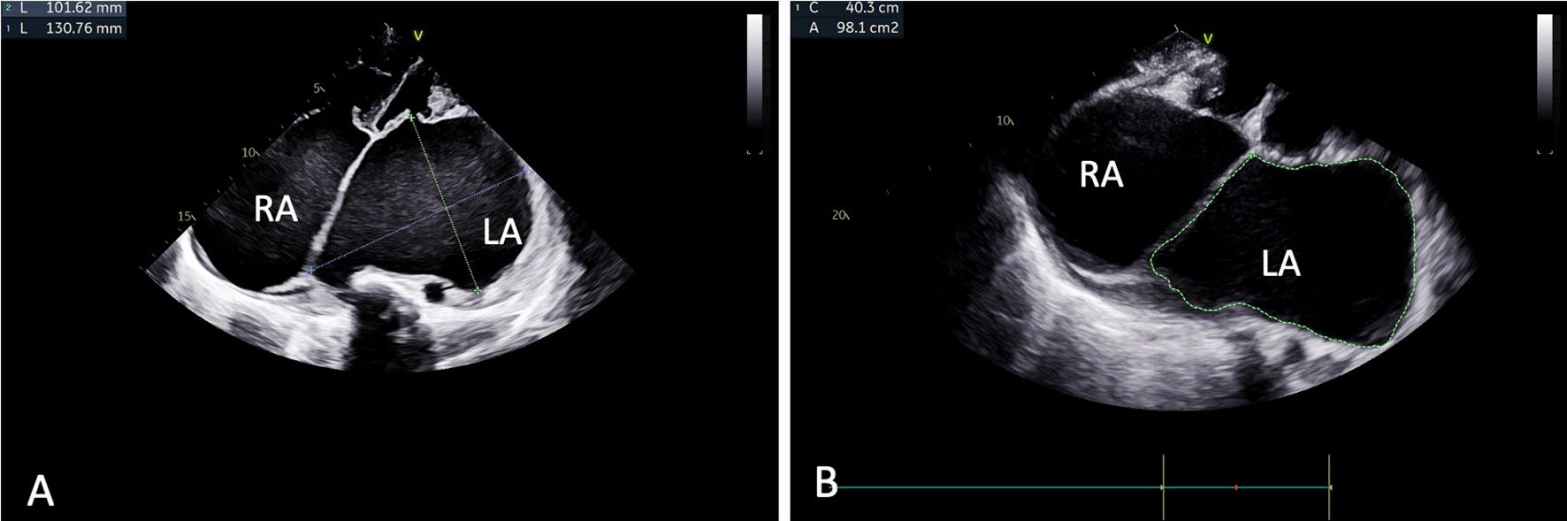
Two-dimensional echocardiography shows giant left atrium with dimensions of 13.0 × 10.1 cms (A) and area of 98.1 cm^2^ (B)
